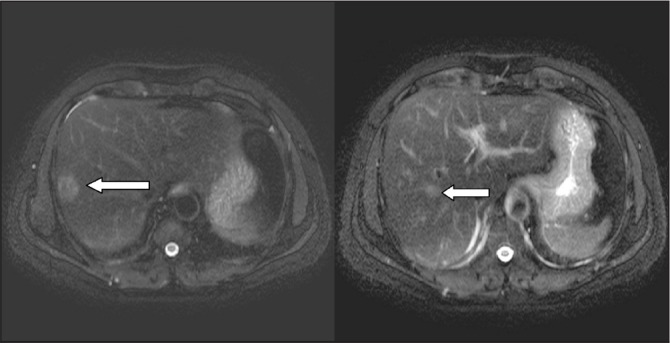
Magnetic resonance imaging showing liver metastasis in segments V and VII/VIII of the right lobe of the liver
